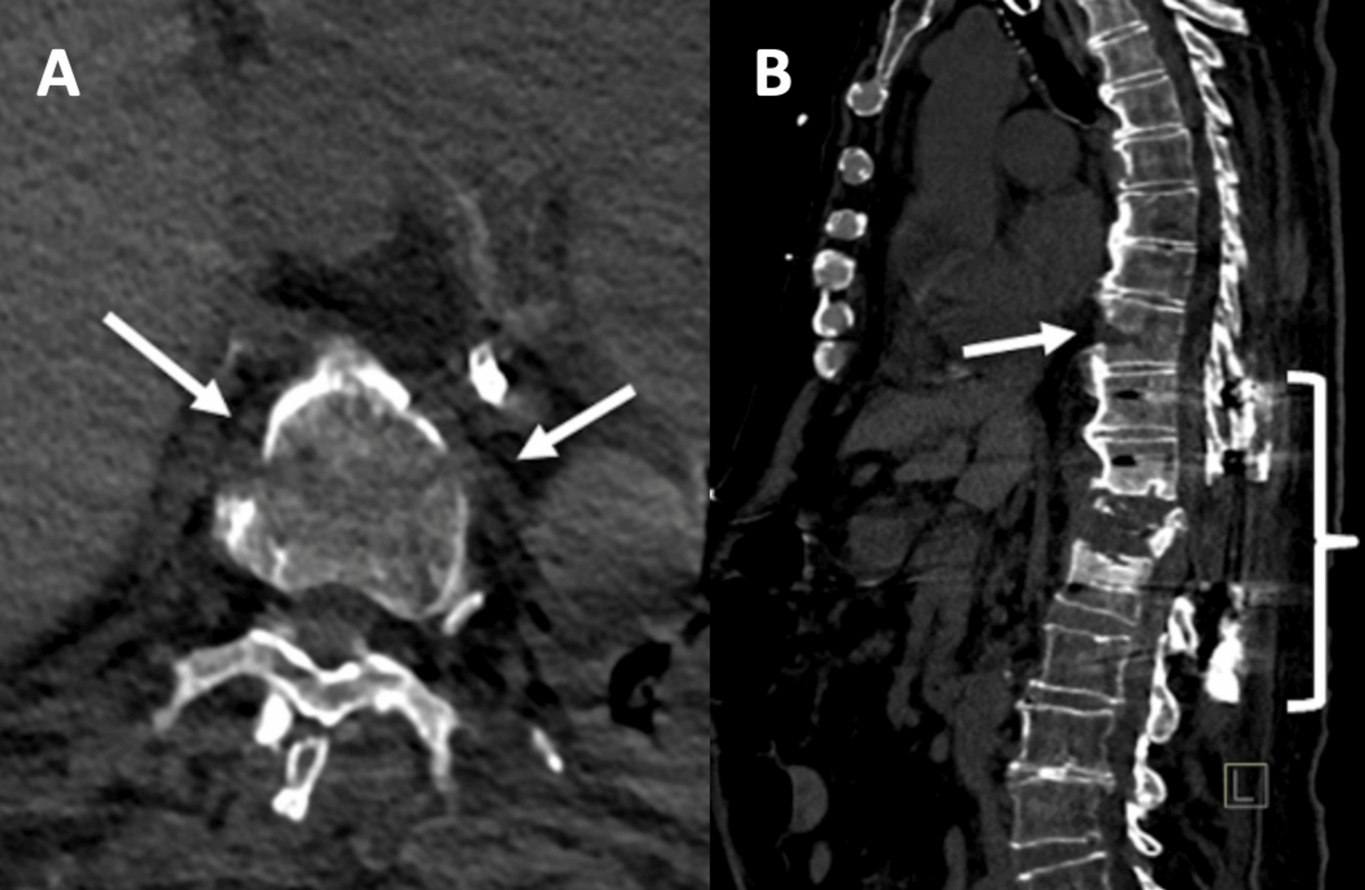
Postoperative CT thoracic spine in the bone window. Postoperative CT thoracic spine in the bone window with axial (A) and sagittal (B) planes for this 79-year-old man, acquired two weeks postoperatively, demonstrating a T9 vertebral body anterior cortex fracture (white arrows) with extension to the inferior endplate, hemorrhage along the fracture line with soft tissue density within the cavity of the fracture space, and T10-L2 fusion hardware (white brackets) surrounding the T12 pathologic compression deformity.
